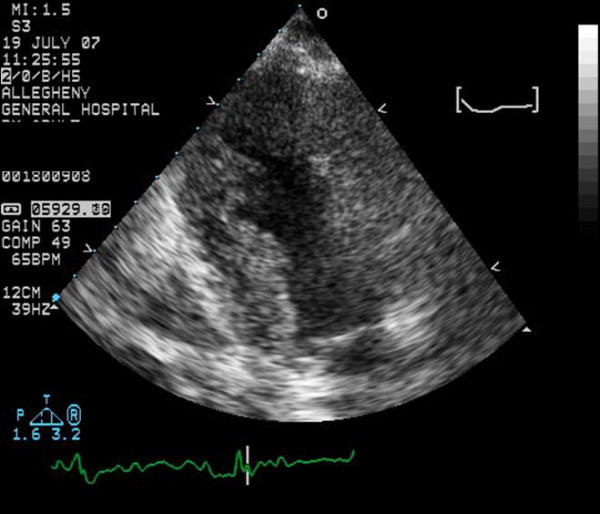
A two chamber view of the transthoracic echocardiogram demonstrating myocardial thickening and slightly irregular endocardial margins. There are no nodular deposits seen on this image.
